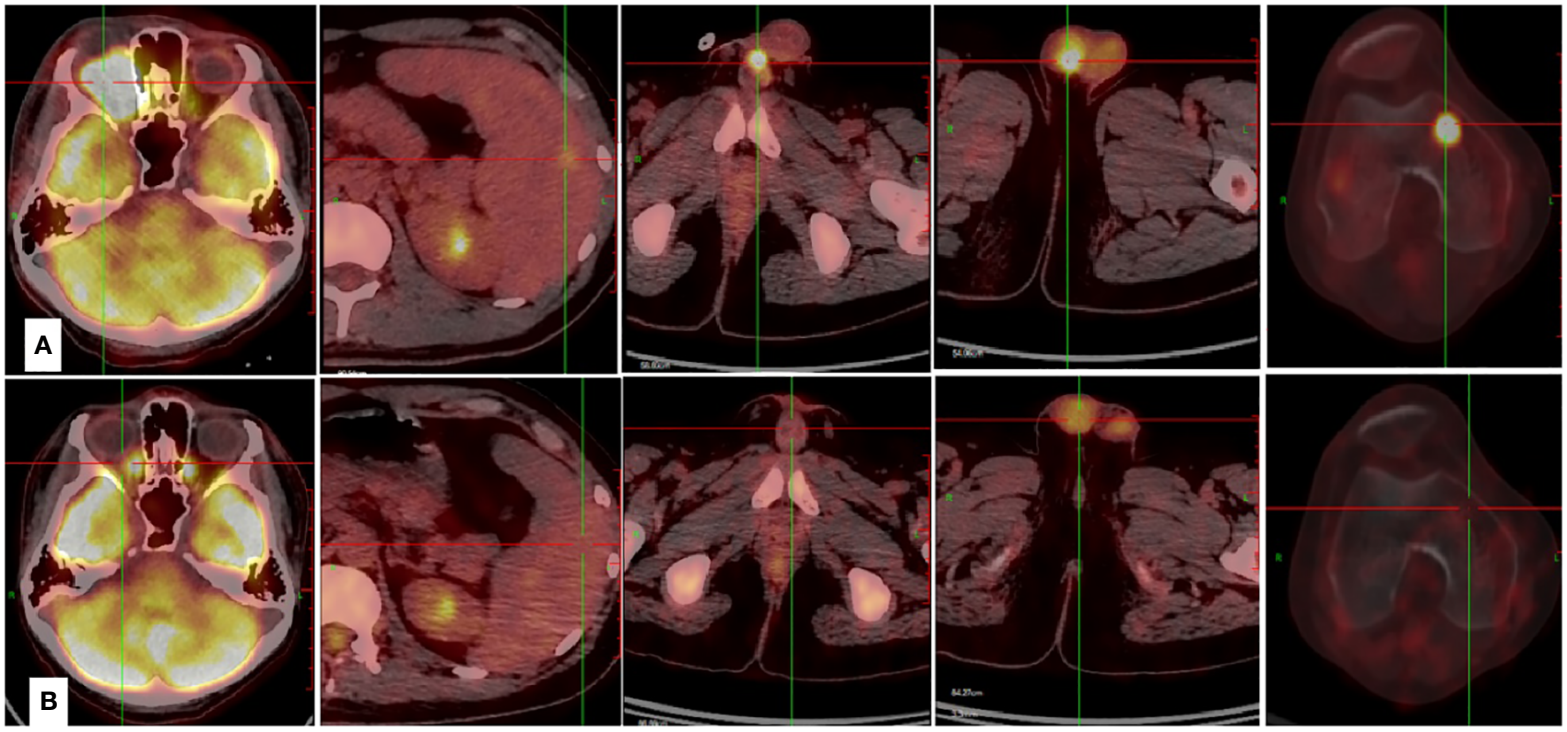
Case presentation of the efficacy achieved by Lipo-MIT based treatments. (A) The PET-CT scan demonstrated wide dissemination of NKTCL before the use of Lipo-MIT based treatment. (B) The PET-CT scan confirmed complete remission of NKTCL after four cycles of Lipo-MIT based treatment.
